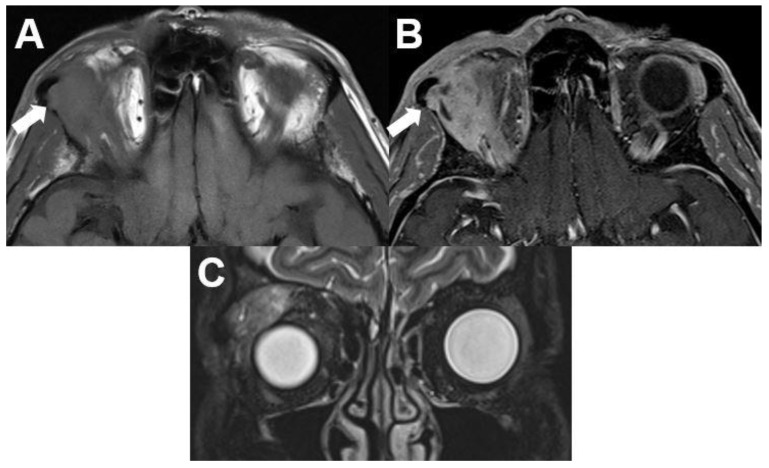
Carcinoma of the lacrimal gland.* MRI of a 59-year-old male patient with a biopsy-proven poorly differentiated carcinoma of the right lacrimal gland. T1-weighted axial images show an ill-defined mass of the right lateral cranial orbit with signal characteristics similar to surrounding muscles (A) and contrast enhancement (B). The adjacent zygomatic bone is infiltrated (arrow). On T2-weighted images of the coronal view, the tumor shows an inhomogeneous signal (C).
